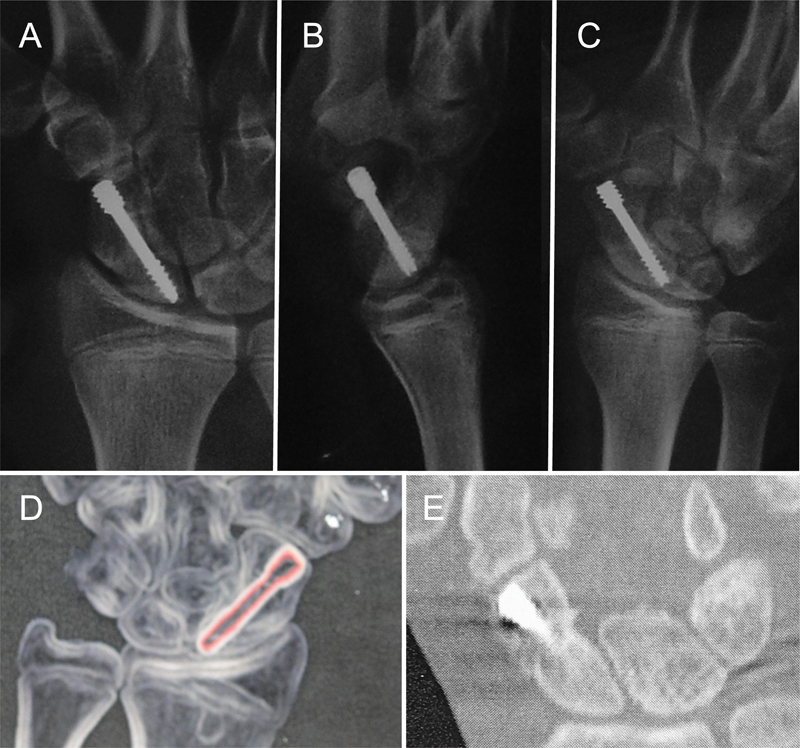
Consolidation of pseudarthrosis after five weeks of evolution. Observed by radiographs (A, B and C) and computed tomography (D and E).
